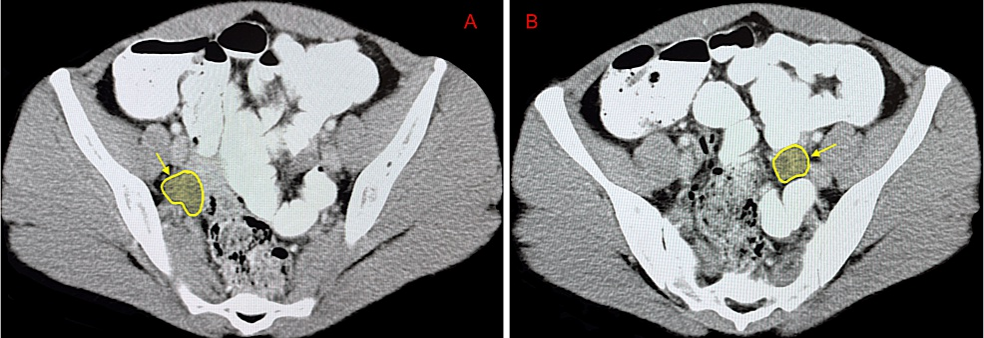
CT of the abdomen and pelvis with contrast revealing bilateral ovarian cysts (yellow arrow) in patient 2 A: The right ovarian cyst, measuring 2.4 cm. B: The left ovarian cyst, measuring 1.6 cm. CT: computed tomography
